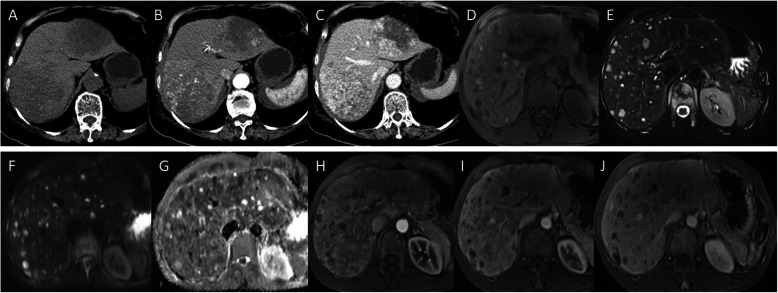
Typical cases of PHA. A 75-year-old female patient with PHA. The unenhanced CT image shows an irregular, ill-defined hypoattenuated hepatic mass (a), marginal patchy enhancement on the arterial phase (b), and progressive, gradual filling enhancement on the portal venous phase (c). A 43-year-old male patient with PHA. The MR images show hypo/hyperintense diffuse lesions on T1WI (d), hyperintense signals on T2WI (E), hypo/hyperintense signals on DWI (f) and the apparent diffusion coefficient (ADC) map (g), marginal patchy enhancement on the arterial phase (h), and progressive, gradual filling enhancement on the portal venous phase (i) and delayed phase (j)
